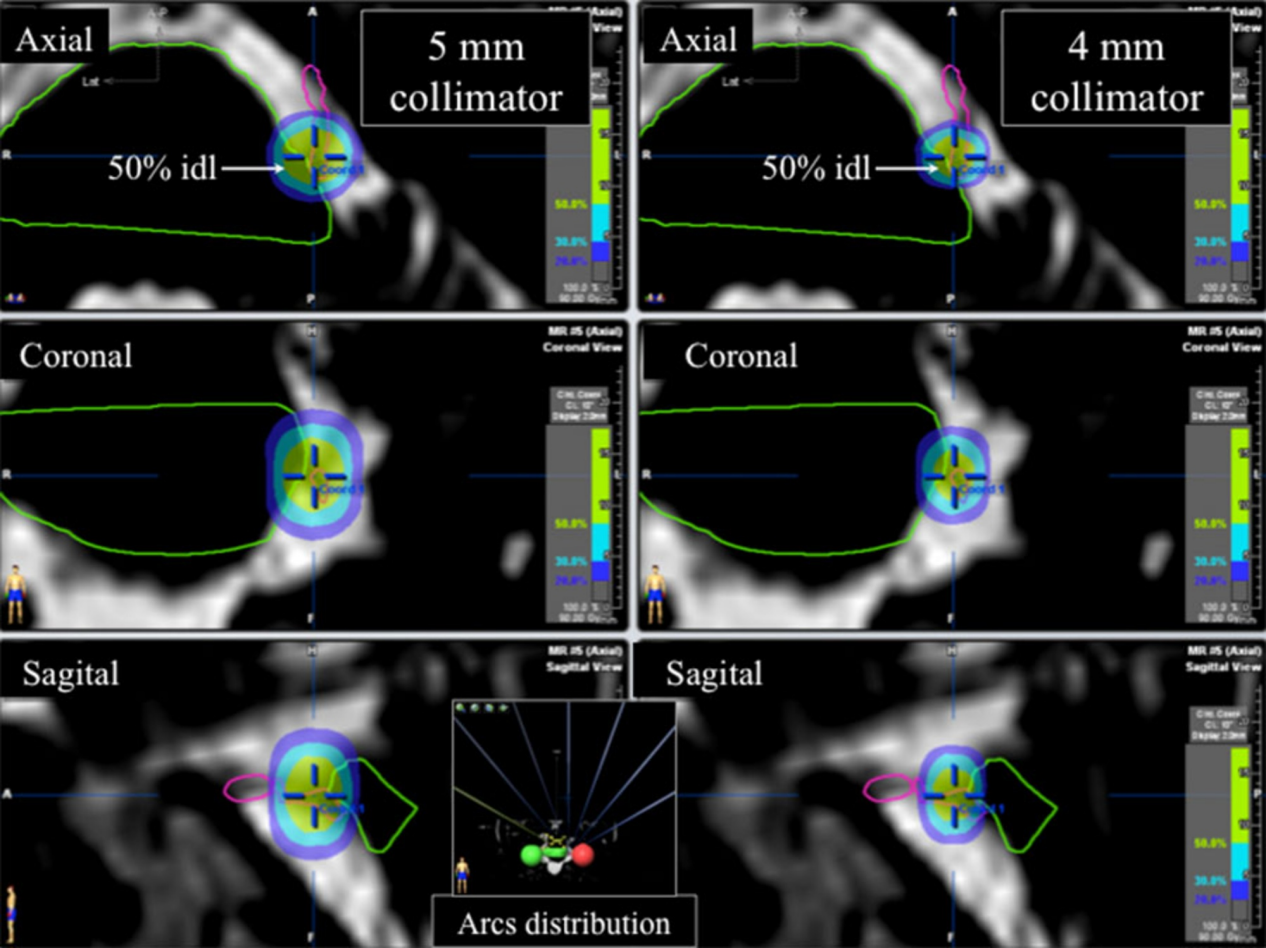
Radiosurgery plan Dose distribution of radiosurgery plans shown in axial, coronal and sagittal plans on CISS MRI sequence: a 5-mm collimator plan is shown in the left columns, while a 4-mm collimator is shown in the right columns. The arc distribution is presented in the lower central portion of the figure. The seven equally distributed arcs are displayed in coronal view.
